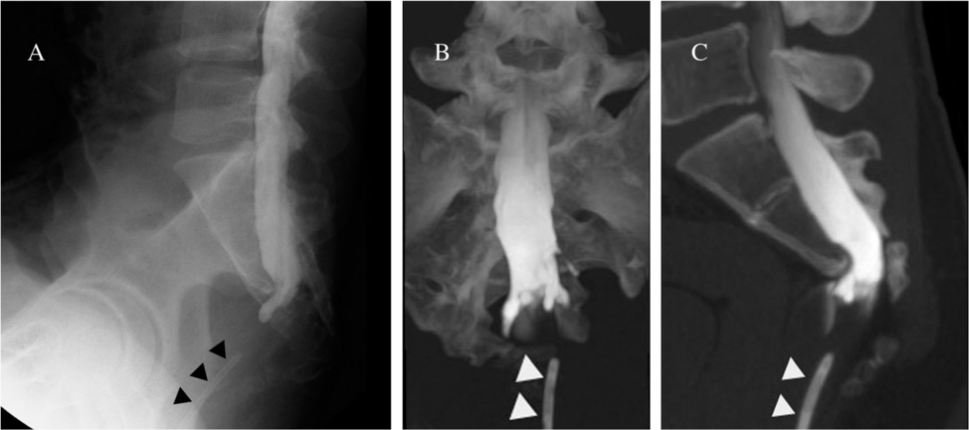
Myelography and postmyelography computed tomography findings. (A) Sagittal myelography showed no apparent communication between the presacral mass and the thecal sac. (B, C) Coronal and sagittal postmyelography computed tomography demonstrated that the terminus of the thecal sac formed some processes. The drain (black (A) and white (B, C) triangles) was placed via a perianal insertion.
